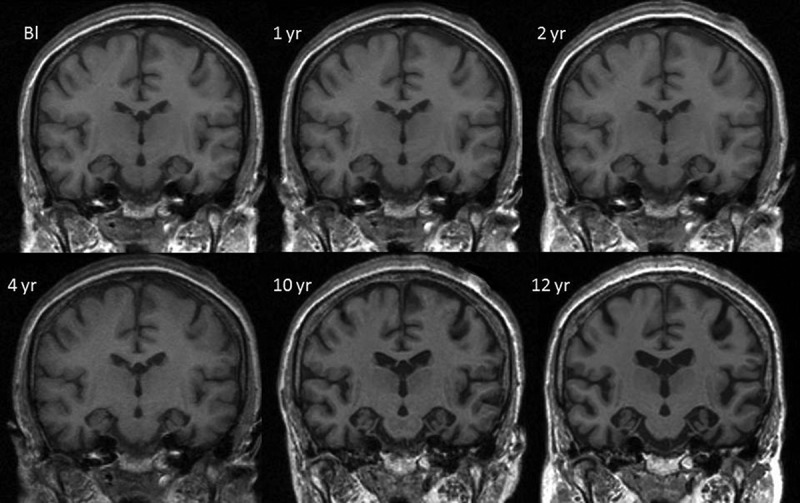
Mid-temporal axial volumetric T1-weighted MR images acquired at initial presentation and subsequent repeat visits (1-, 2-, 4-, 10-, and 12-year follow-up). All repeat images have undergone 12 degrees of freedom registration to spatially align them to the baseline (Bl).
